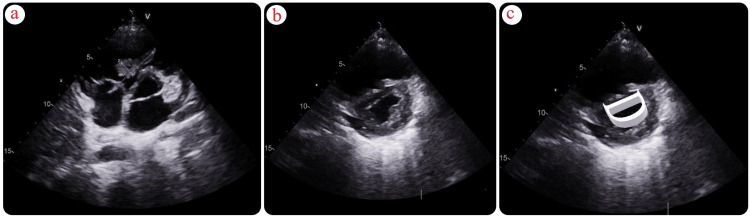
Transthoracic Echocardiogram: Right Ventricular Findings (a) Transthoracic echocardiogram (parasternal, short-axis view, aortic valve level) demonstrating in-transit thromboemboli in the right atrium (RA) crossing the tricuspid valve (TV) into the right ventricle (RV). (b,c) Transthoracic echocardiogram (parasternal short-axis view at midventricular level) demonstrating right ventricle (RV) dilatation and interventricular septal flattening (D-shape).
